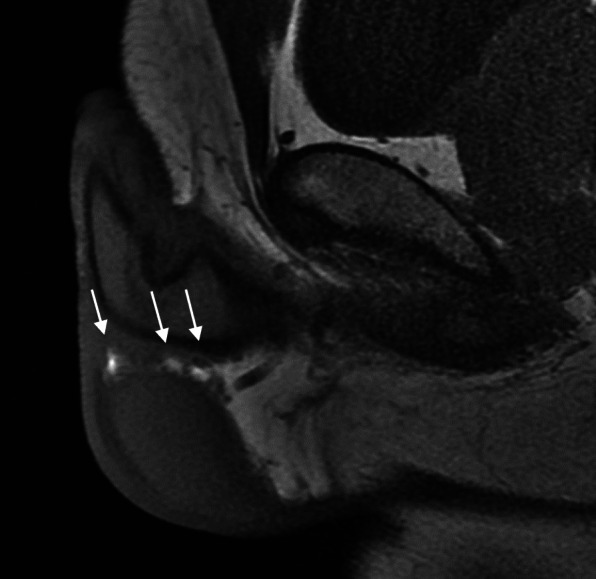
Sagittal T1-weighted post contrast MRI of penile nodules. Sagittal T1-weighted post contrast MRI of penile nodules displaying low signal intensity (arrows)
